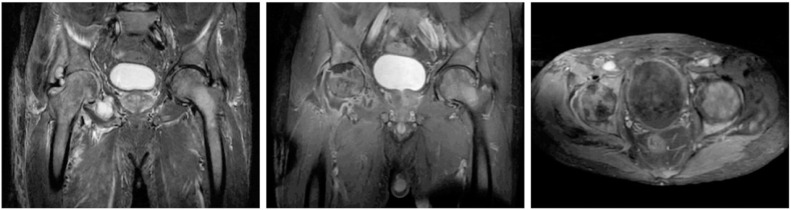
Magnetic resonance imaging of the pelvis showing avascular necrosis of the right femoral head, chondral thinning and narrowing of the right hip joint space, large right hip joint effusion with distension of the articular recesses and capsular enhancement after contrast administration; in addition to edema in the gluteal muscles and the proximal right thigh, along with signs of bone marrow infiltration of the pelvis, sacrum, studied vertebral bodies, and bilaterally of the femurs.
